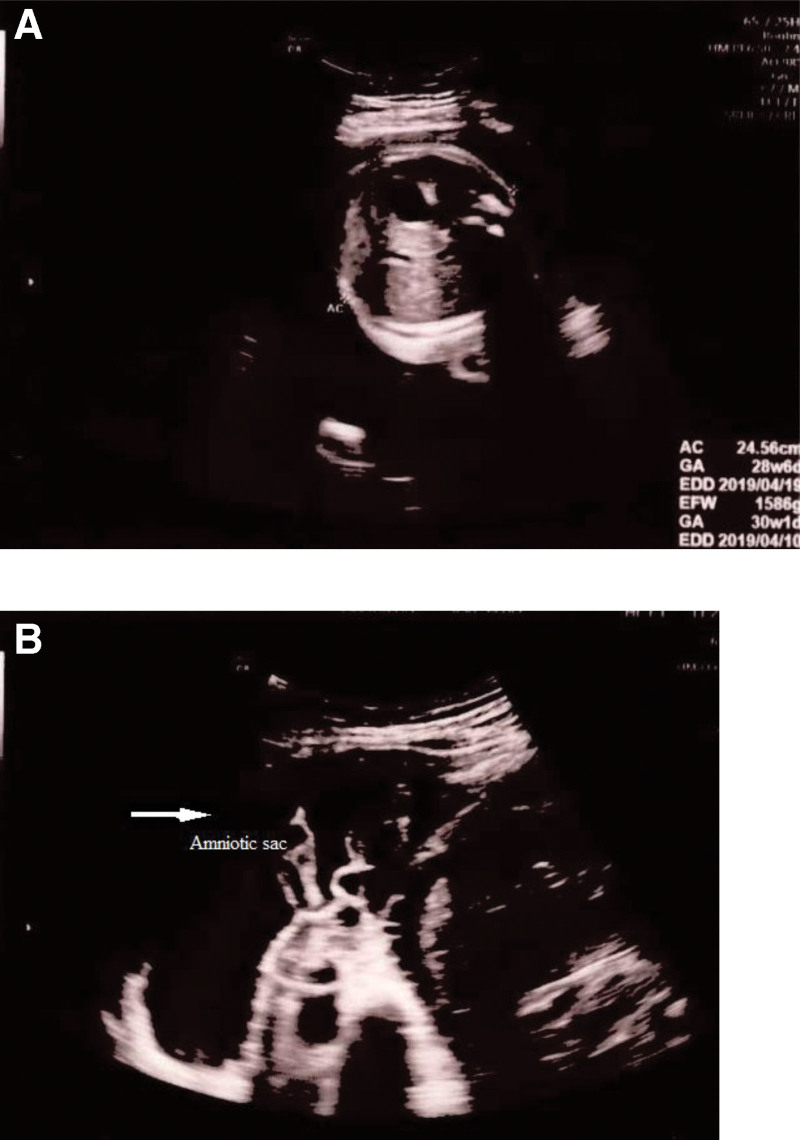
Ultrasound examination before and after uterine rupture. (a) Absence of abnormal findings related to amniotic fluid volume and placentation. (b) Protruding amnionic sac (arrow) observed through a large uterine defect.
